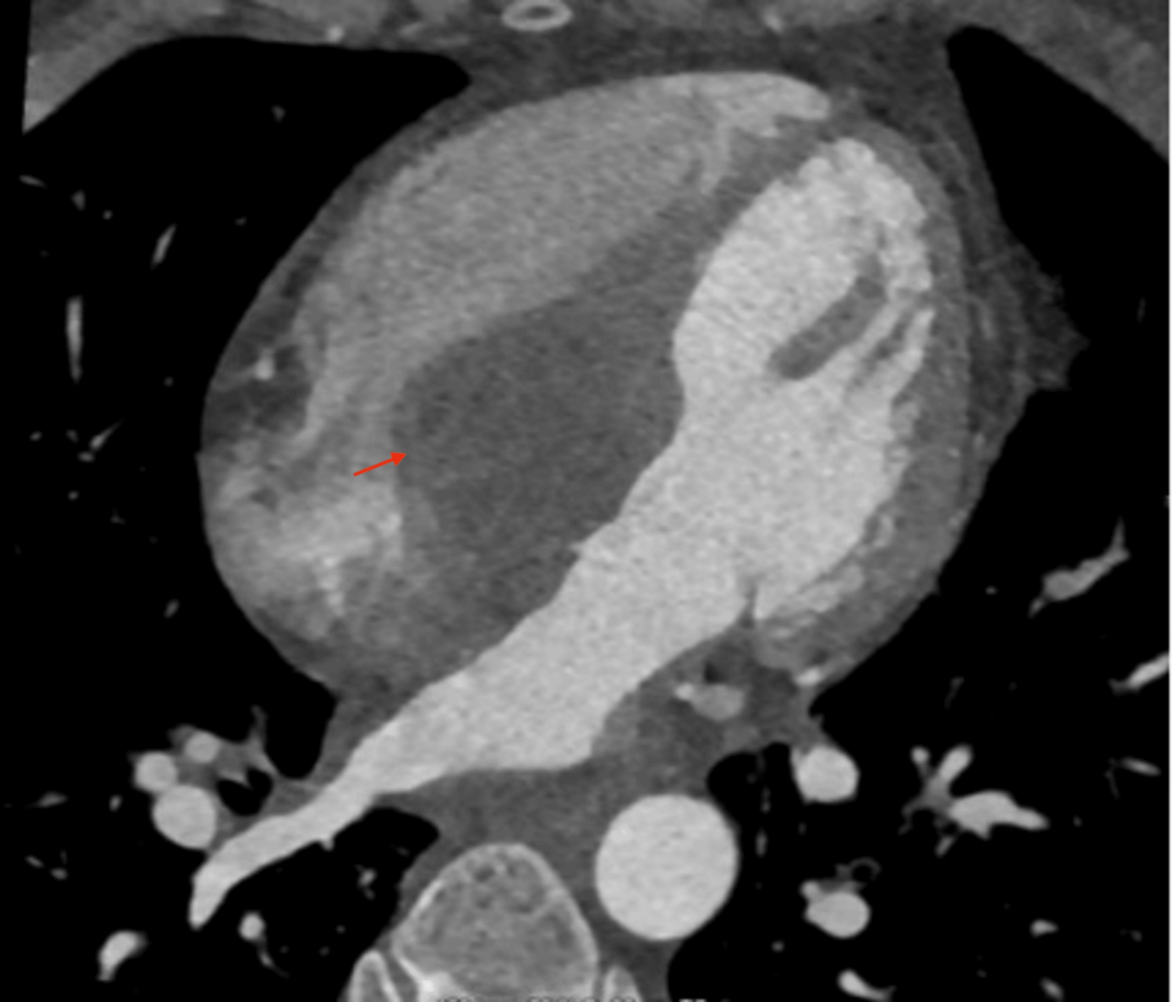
Gated coronary CTA showed a hypoattenuating structure located in the basal interventricular septum, adjacent to the tricuspid valve, with pre-contrast HU of 3 HU and post-contrast HU of 40-60 HU, suggesting a fluid-based mass with possible vascularity CTA: computed tomography angiography
